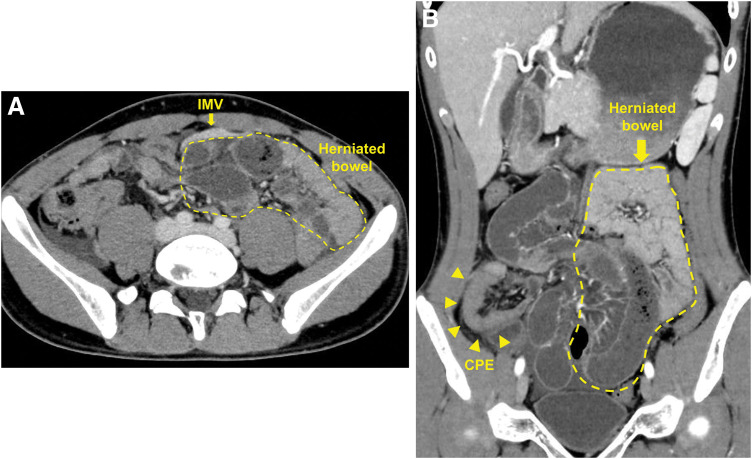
Preoperative contrast-enhanced CT findings. (A) Axial view. A partially dilated loop of the small intestine was observed herniating into the left side of the abdomen and pelvis (area enclosed by dashed lines), positioned dorsally to the abnormally routed the IMV (indicated by the arrow). These findings are indicative of left paraduodenal hernias. (B) Coronal view. The complex pathology is depicted. Another segment of the small intestinal loop is encased within a thin accessory peritoneum (indicated by arrowheads) in the right lower abdomen, a finding consistent with CPE. Additionally, a large hernia was observed in the left paraduodenal space. CPE, congenital peritoneal encapsulation; IMV, inferior mesenteric vein
